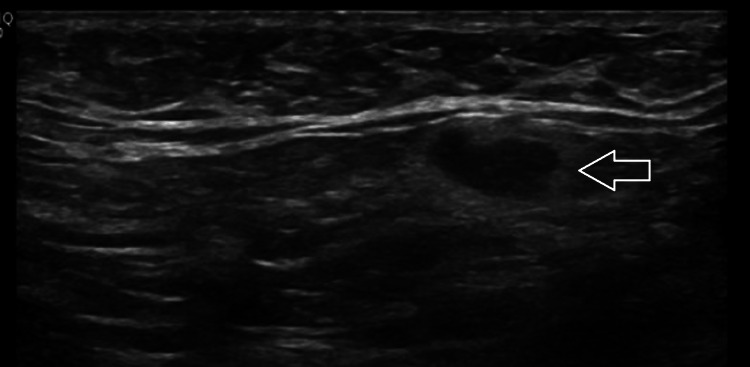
Ultrasound of the left axillary lymph node showing cortical thickness at the upper limit of normal
